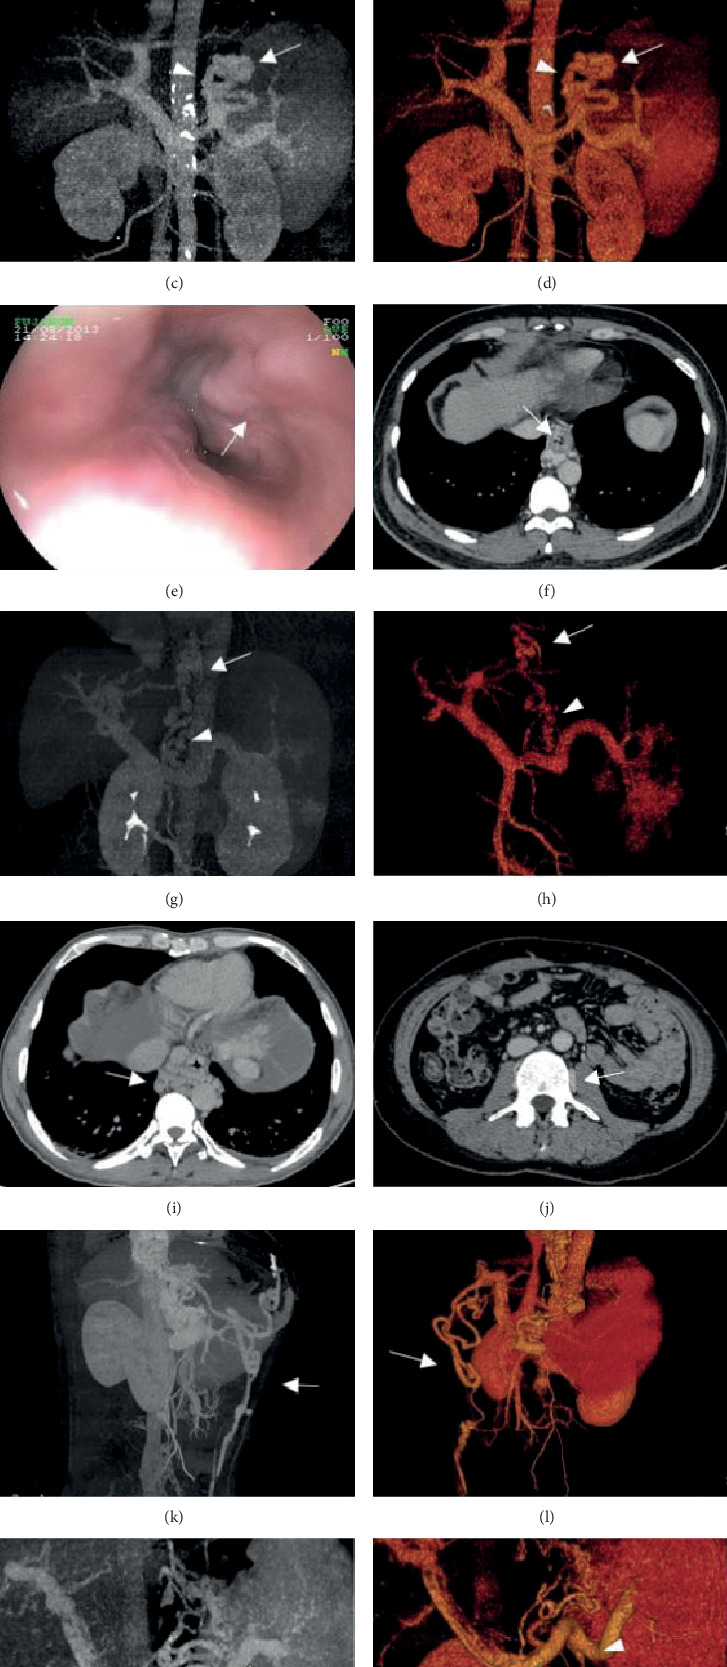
Computed tomography portal venography (CTPV) is comparable to endoscopy in the detection of gastroesophageal varices, and it is superior to endoscopy in the evaluation of the whole portal system. Severe gastric varices were clearly revealed by both (a) endoscopy and (b) CTPV. The (c) CT-MIP images and (d) CT-VR images demonstrate gastric varices (arrow) originating from the short/posterior gastric vein (arrowhead). Esophageal varices are depicted by (e) endoscopy and (f) CTPV. The (g) CT-MIP images and (h) CT-VR images reveal the left gastric vein as the inflowing vessel (arrowhead) of the varices (arrow). Axial computed tomography images show (i) paraesophageal varices and (j) paravertebral varices. Reconstructed images show the (k, l) tortuous paraumbilical vein and (arrow, m and n) spontaneous splenorenal shunt connecting the (curved arrow, m and n) left renal vein and (arrowhead, m and n) splenic vein.
